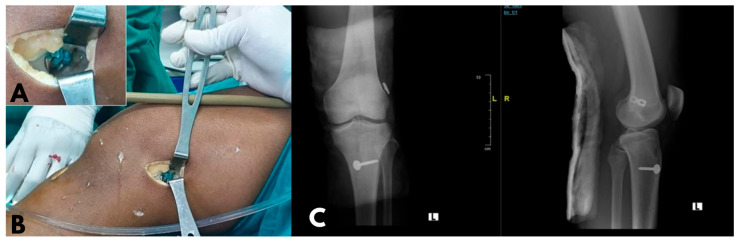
Anterior cruciate ligament reconstruction using a modified implant. (A) Modified reconstruction plate being placed on the femoral site. (B) A well-fixed modified implant at the femoral site is shown. (C) An anterior–posterior–lateral knee radiograph post-operation using the modified implant is shown.
